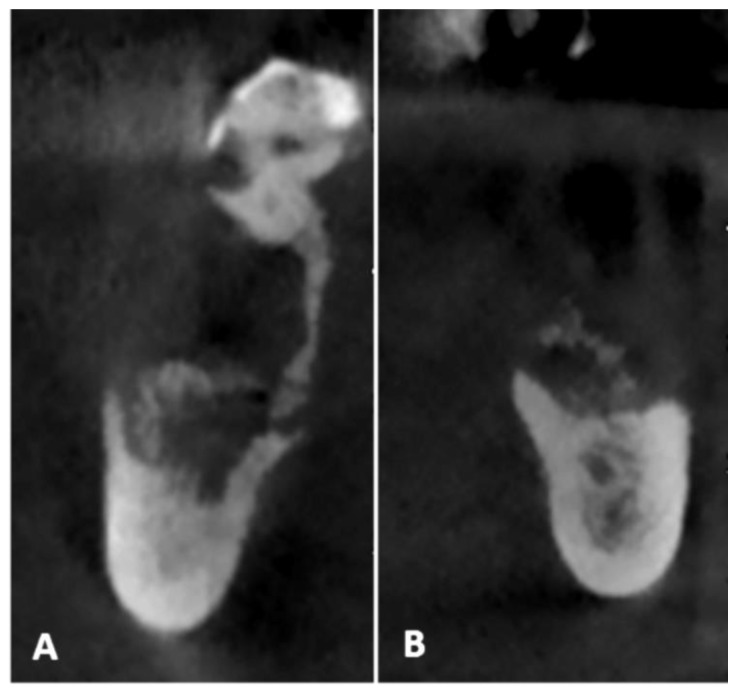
The presence of altered bone morphology on the preoperative CBCT (A,B), with the involvement of the right-sided mandibular canal with loss of root continuity (A).
